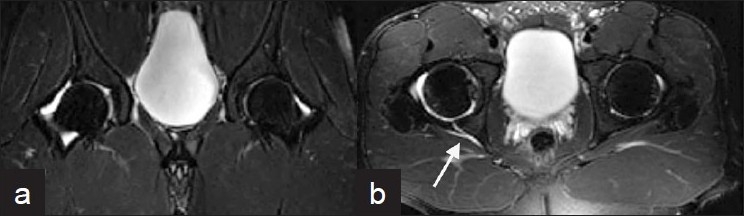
MRI performed three hours after injury. (a) Coronal fast spin echo T2-weighted image with fat saturation shows a small right hip effusion and no marrow abnormality. (b) Axial fast spin echo T2- weighted image with fat saturation shows a right posterior labral tear (arrow) with fluid dissecting posterior to the acetabulum. (Images obtained on a 1.5T General Electric MR scanner)
